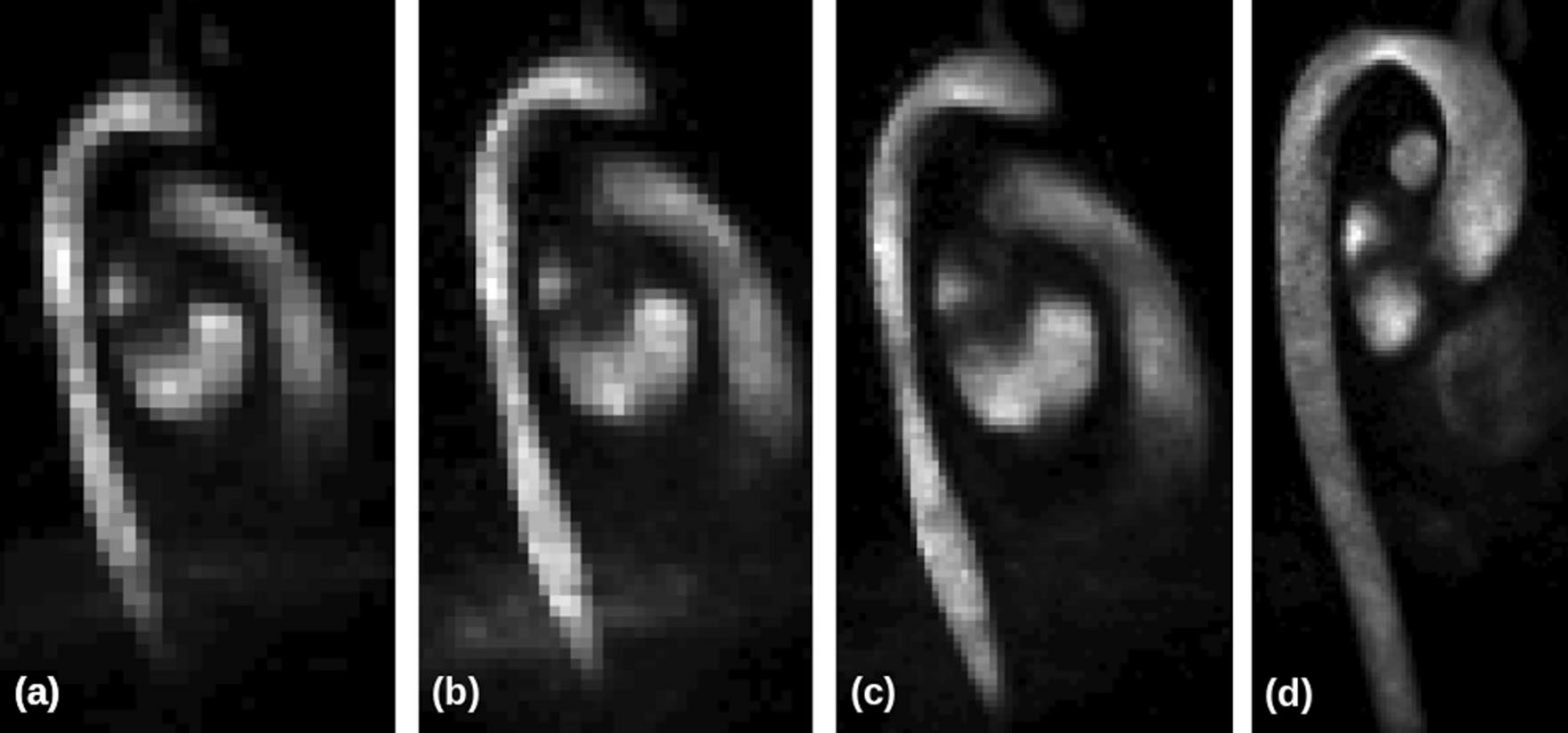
4D-flow MRI images of the thoracic aorta at the same location in the sagittal plane, taken at four varying spatial resolutions ($$x \times y \times z$$
